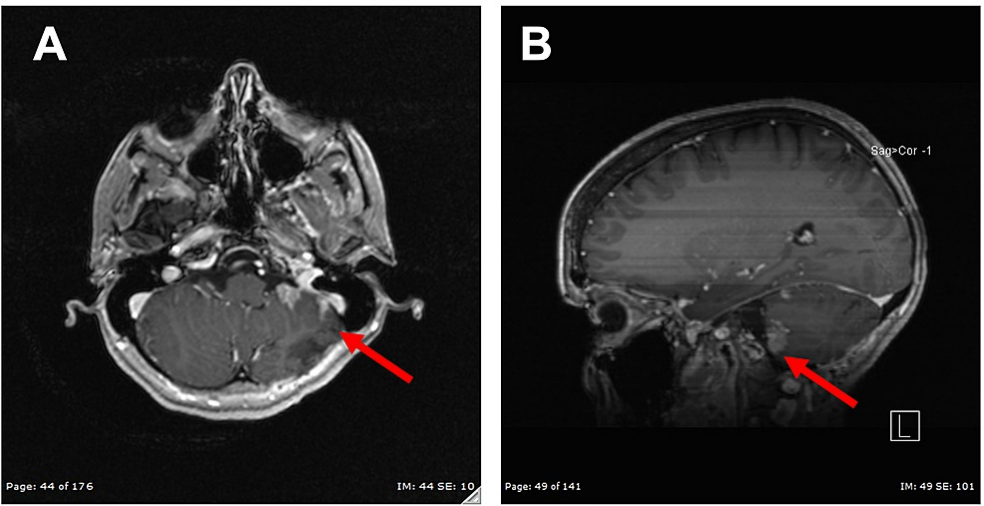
MR Images Showing Growth of Two Previous Lesions (A) Axial T2-weighted MR images demonstrating growth of two previously noted lesions as well as appreciation of new lesion within the superior anterior aspect of the left cerebellum (red arrow). (B) Sagittal T1-weighted MR images with gadolinium contrast demonstrating growth of two previously noted lesions as well as appreciation of new lesion within the superior anterior aspect of the left cerebellum (red arrow).
